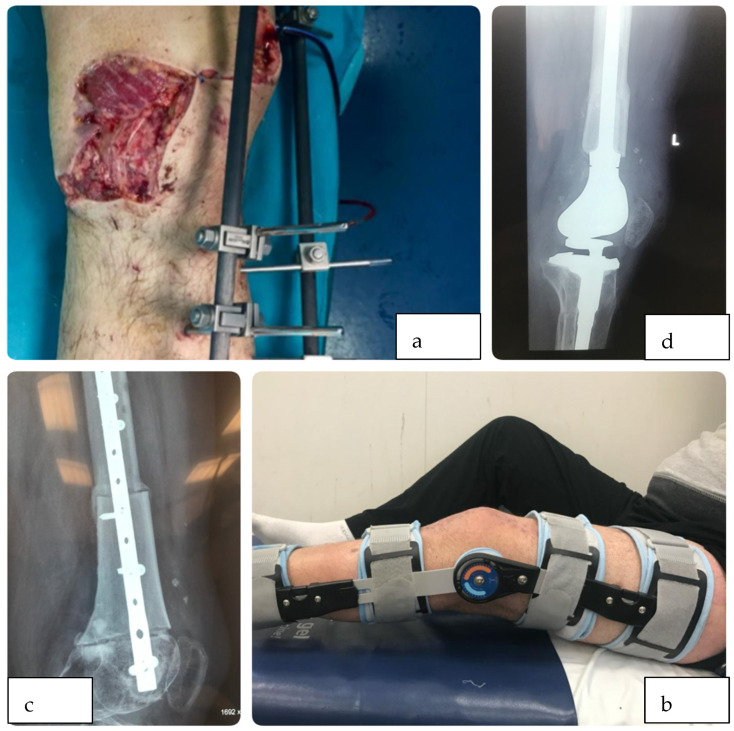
Case 2: Postoperative course of a 30-year-old male with a massive defect, complicated by nonunion and ultimately treated with conversion to arthroplasty. (a) Clinical presentation of the massive bone defect and soft-tissue loss managed with a temporary joint-spanning external fixator during the initial damage-control surgery in July 2016. (b) Clinical photograph showing the patient fitted with a hinged knee brace following external fixator removal to allow for early joint mobility. (c) Radiograph demonstrating the third surgery with an intramedullary nail and structural allograft. This intermediate stage ultimately failed to achieve distal union, developing a symptomatic pseudarthrosis. (d) Final anteroposterior radiograph demonstrating successful conversion to a total segmental knee arthroplasty, utilizing the proximally integrated allograft for stable stem anchorage in July 2018.
